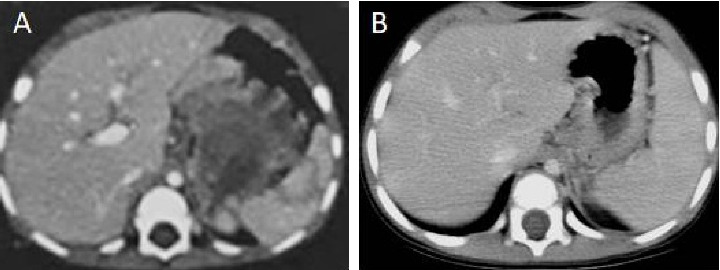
A computed tomography scan three cycles before chemotherapy reveals a large, heterogeneous mass in the gastrohepatic region (A). Significant reduction in lesion size was observed after chemotherapy, with disease now involving the proximal body of the stomach (B).
